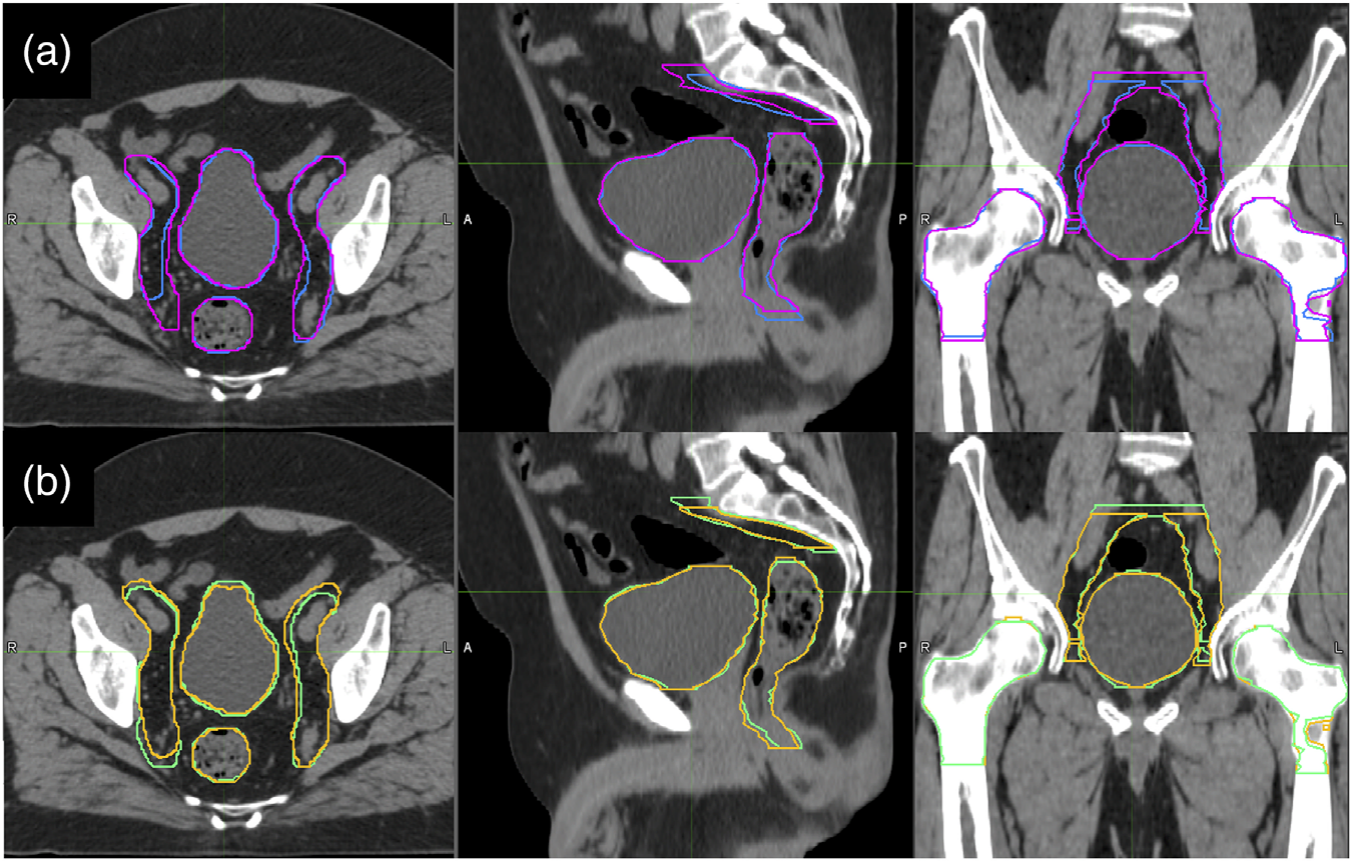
Single radiation oncologist (RO) contours are shown on the computed tomography (CT) images of a test patient: (a) M1 (blue) and M2 (magenta) contours; (b) AM1 (green) and AM2 (yellow) contours
